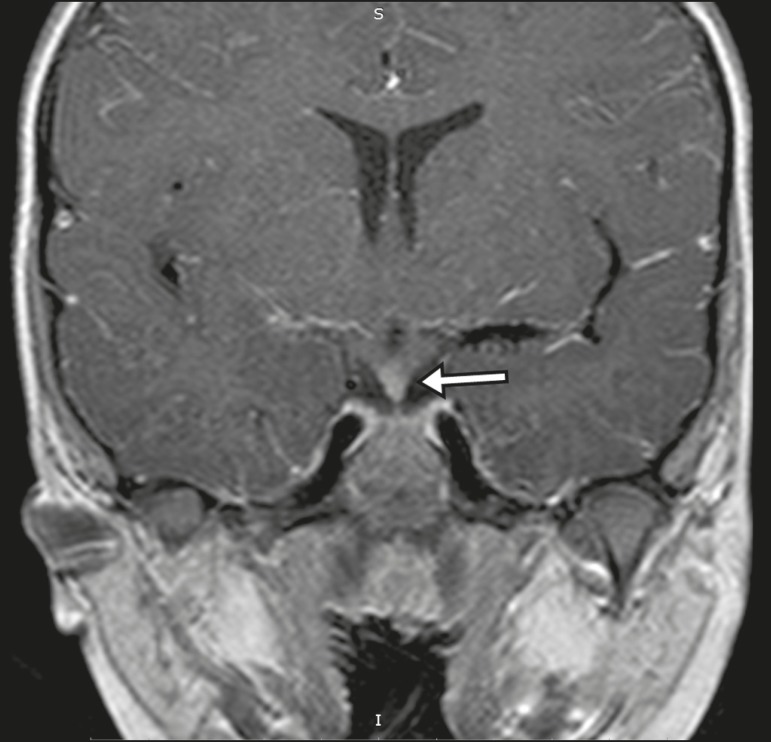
Non-contrast-enhanced coronal T1-weighted MRI sequence, showing
